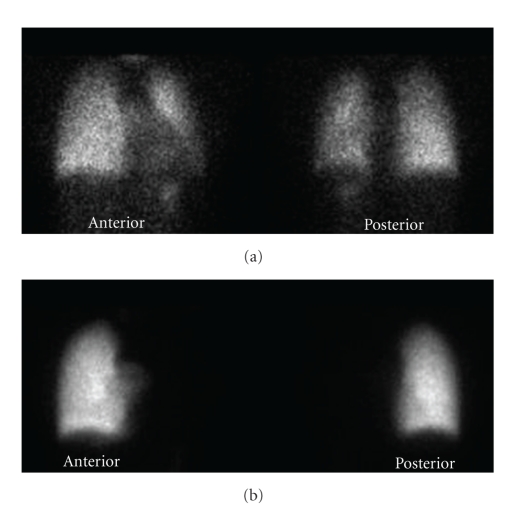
PA chest radiograph shows a small left lung volume with decreased vascular markings.
